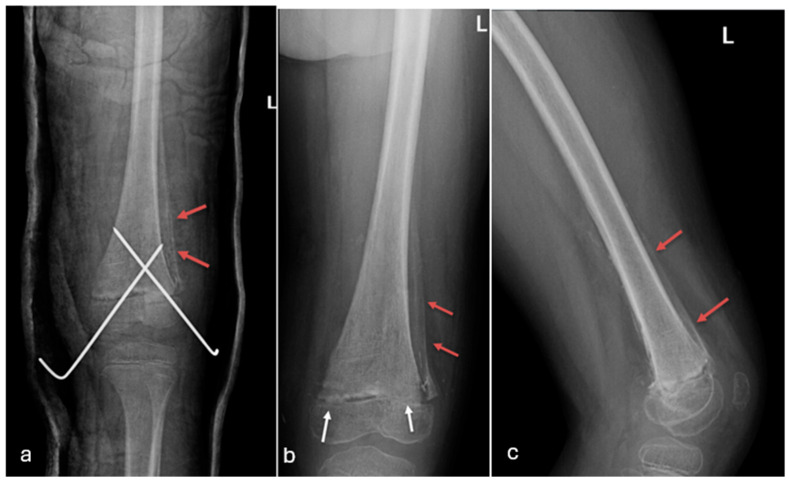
X-ray evaluation: anteroposterior radiograph: (a) one month after the operation, evaluation regarding the stability of fragments, and consolidation is underway; (b) Anteroposterior radiograph two months after operation, morphological irregularities (closing growth plate) were noted within the distal femoral physis (white arrows), as well as revealing signs of periosteal proliferation, likely secondary to the ossification of a post-traumatic soft tissue hematoma (red arrows); (c) Lateral radiograph of the evaluation of the femoral diaphysis.
